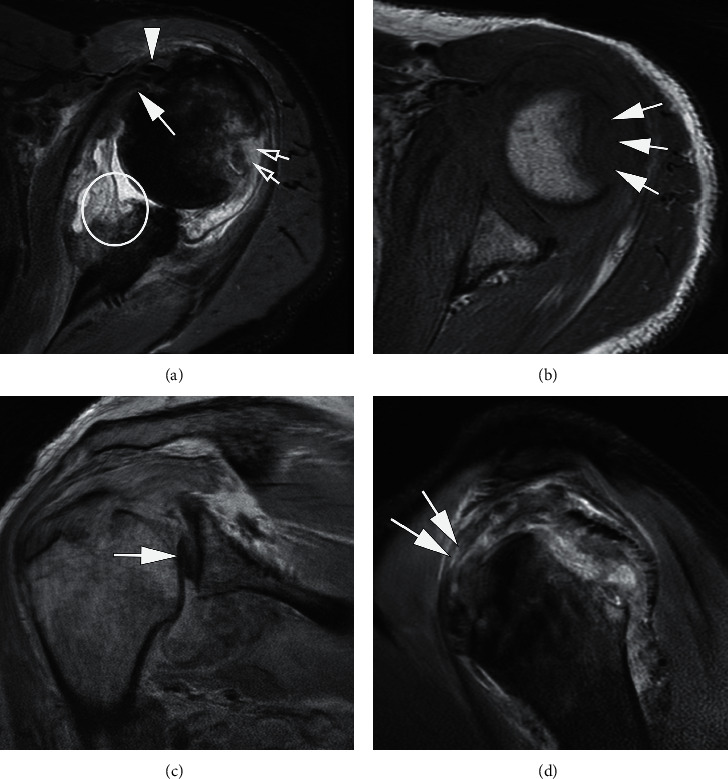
A 67-year-old man with persistent pain after a left shoulder dislocation. Axial T2-weighed FS MRI (a) shows a partial articular surface tear of the subscapularis tendon (large arrow) with mild medial displacement of the long head of the biceps tendon (arrowhead). Also evident are an osseous Bankart lesion (ellipse) and the corresponding Hill–Sachs deformity (two short arrows). The full extent of the Hill–Sachs depression is best appreciated in the axial PD-weighted MRI (b), consistent with recent anterior dislocation. Coronal PD-weighted MRI (c) demonstrates a superior labral tear with the displacement of a portion of labrum (arrow) into the glenohumeral joint space. Sagittal oblique T2-weighed FS MRI (d) shows an intrasubstance tear of the genu of the long head of the biceps (arrows).
